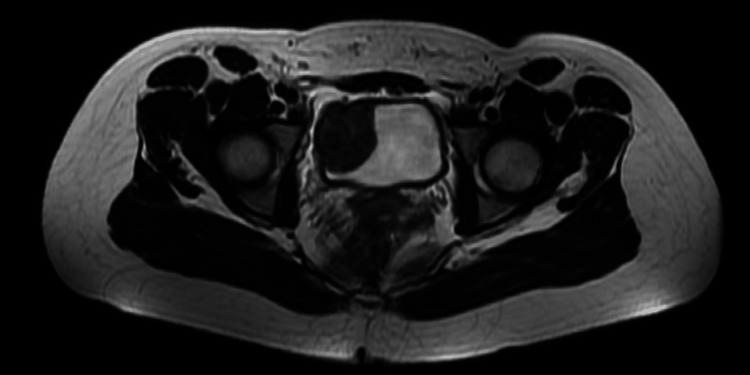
Pre-operative contrast-enhanced pelvic MRI T2 axial
